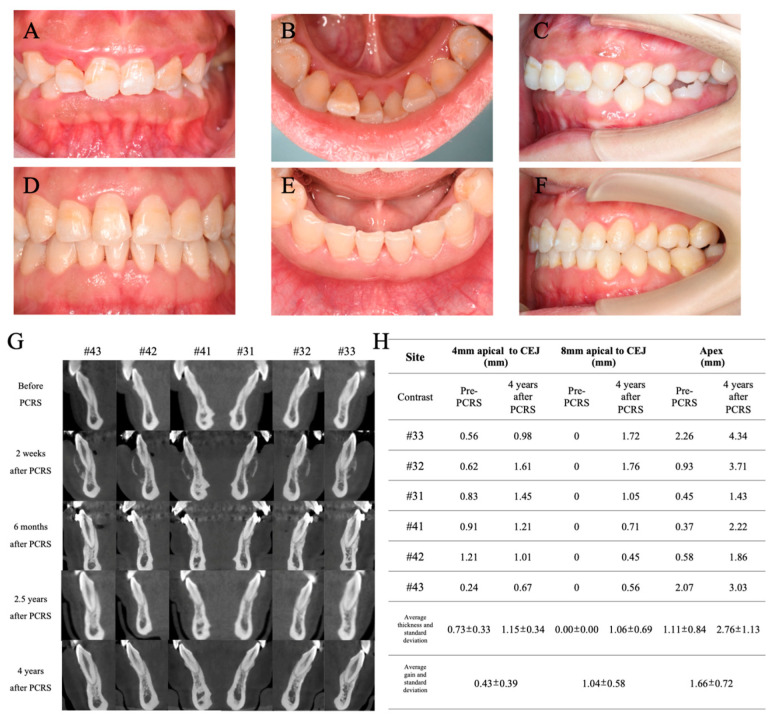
Contrast of clinical photographs and radiographs before and after the combined treatment. Frontal occlusal photograph (A), lingual photograph (B) and lateral occlusal photograph (C) in the first visit. Frontal occlusal photograph (D), lingual photograph (E) and lateral occlusal photograph (F) after 5 years of combined periodontal and orthodontic treatment. (G) Comparison of the sagittal plane in CBCT of the mandibular anterior region before and after PCRS. (H) Comparison of labial alveolar bone thickness between pre-PCRS and 4 years after PCRS at different levels.
